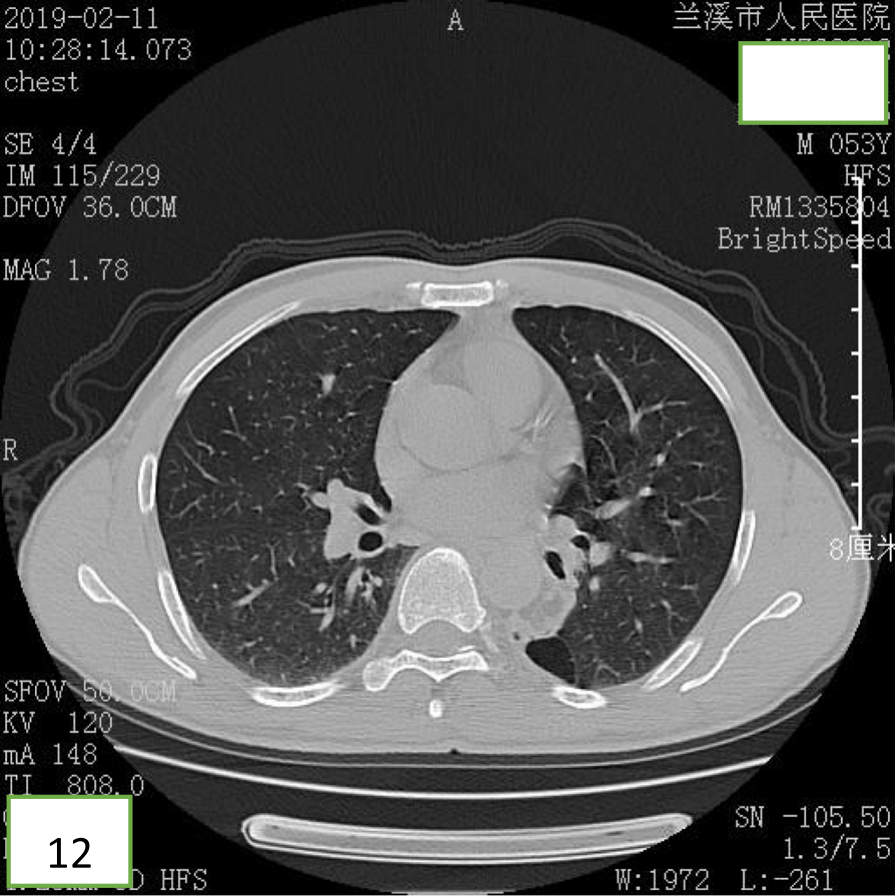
CT revealing that the left main bronchus and the left lower lobe were unobstructed, and that the left lower lobe showed bronchiectasis
